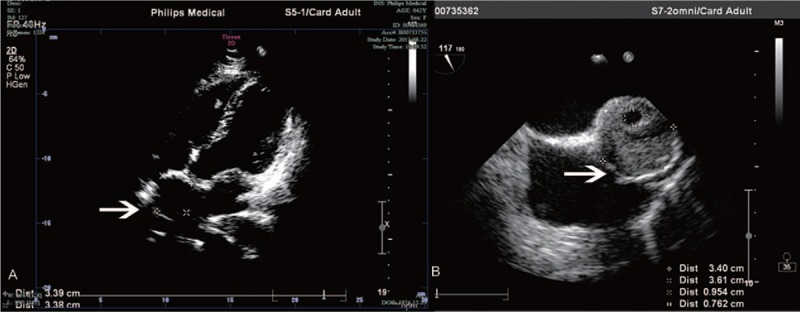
The echocardiography shows a mass in the right atrium. (A) Transthoracic echocardiography and (B) transesophageal echocardiography.
